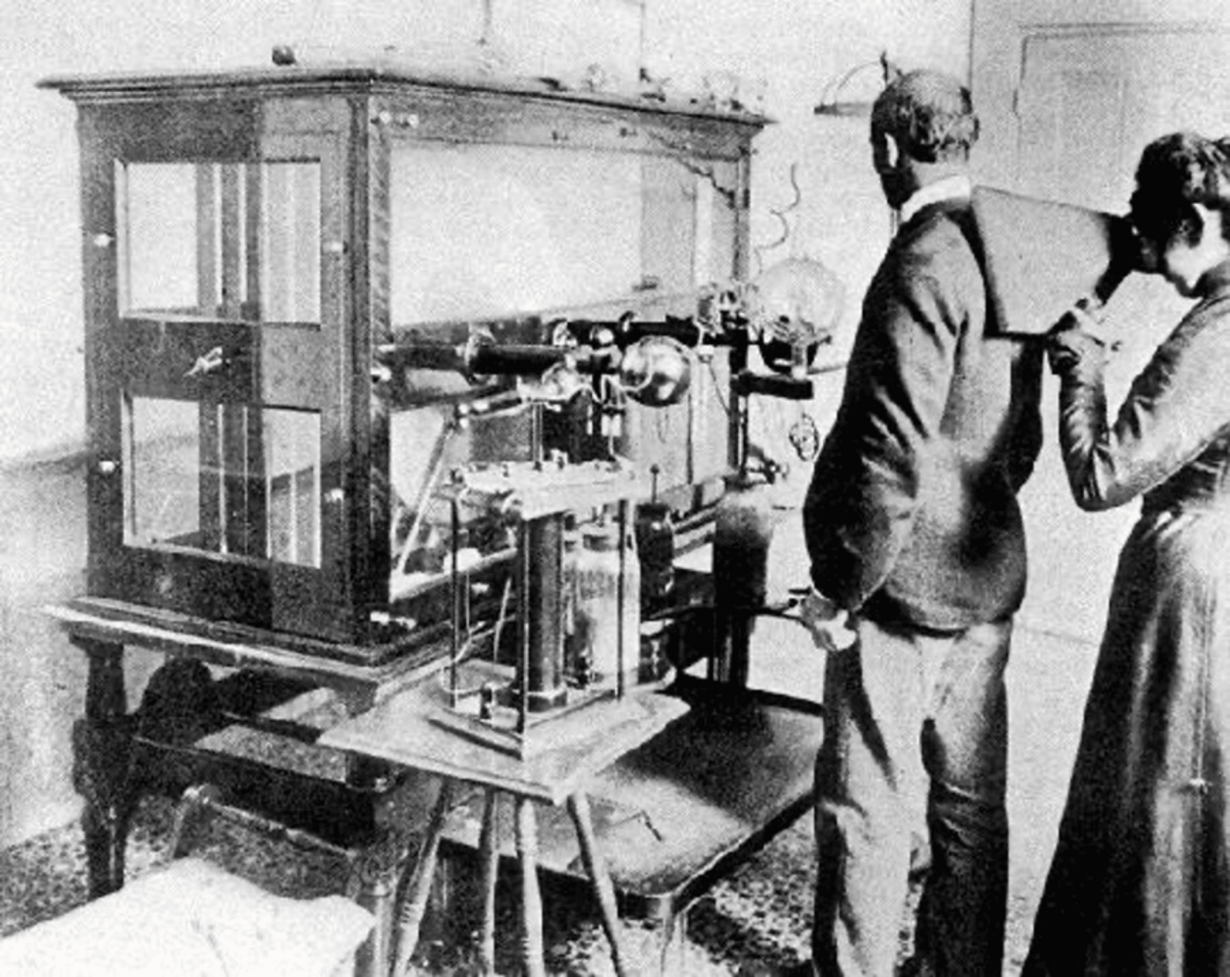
Elizabeth Fleischmann-Aschheim using a portable fluoroscope prior to her death in 1905 From: https://www.foundsf.org/index.php?title=X-ray_Photographer:_Elizabeth_Fleischmann
